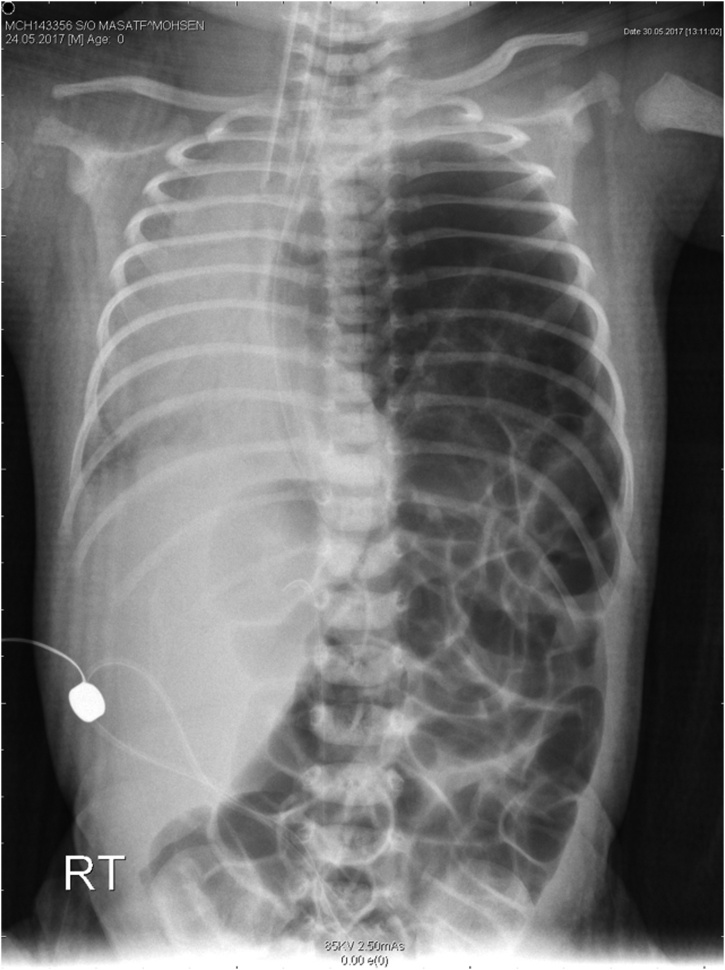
Chest x-ray showing expansion and increasing lucency of the left hemithorax with bowel loops seen within the left hemithorax and mediastinal shift to the right side.
